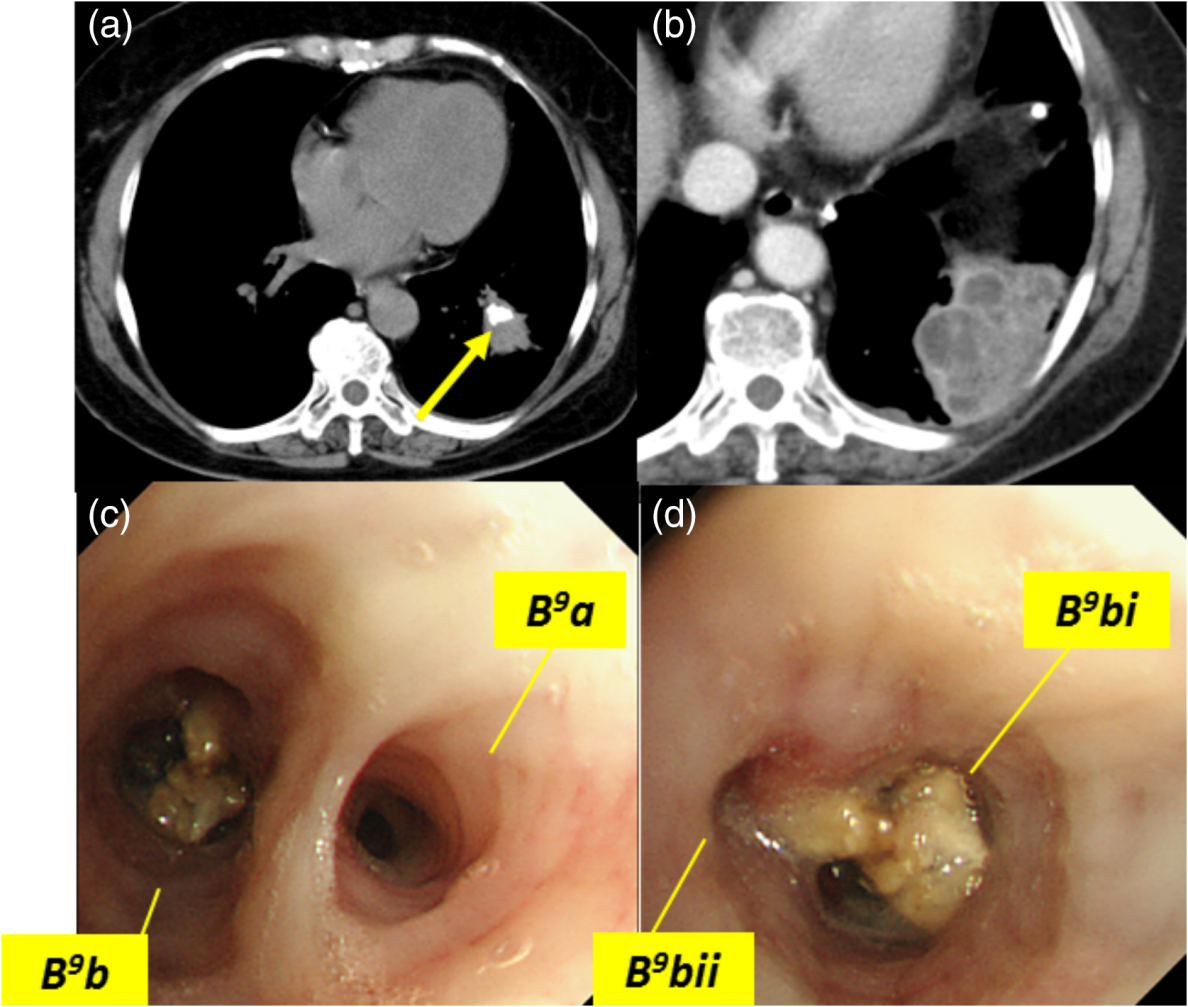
(A) Chest computed tomography (CT) showing calcified nodules distributed in the left lower lobe. (B) Chest CT showing calcified nodules in the left lower lobe combined with consolidation. (C) Bronchoscopic examination showing left lower lobe and basal segmental bronchial abscess.
